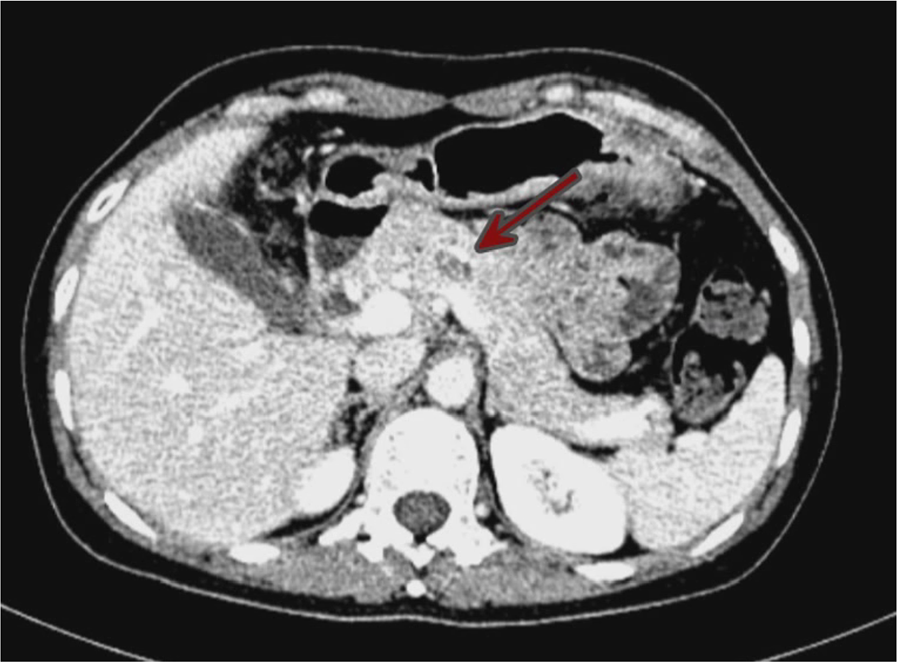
CT demonstrated a 13 × 14-mm, low-density mass in the pancreatic neck.
